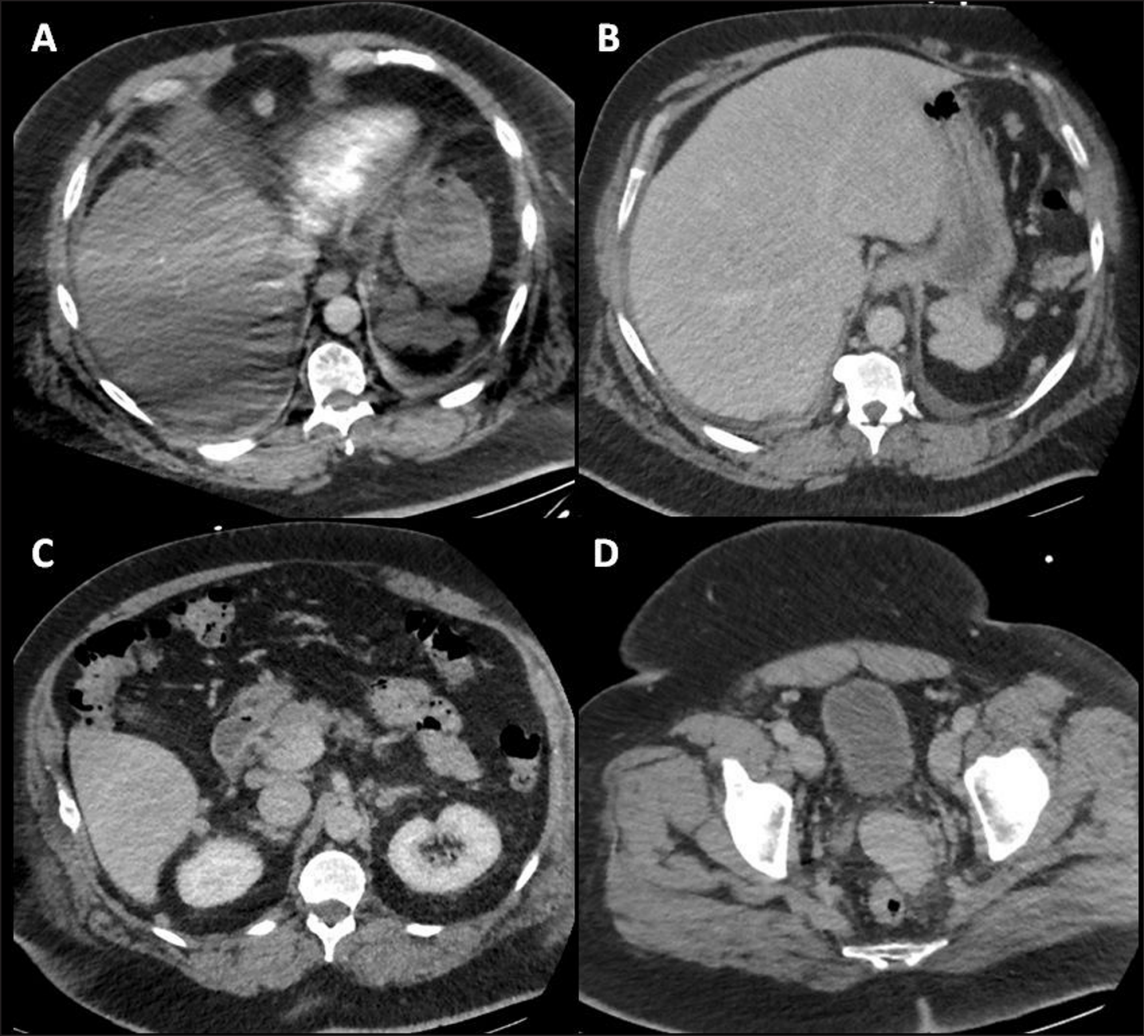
CT pulmonary angiography, arterial phase, axial slice (A) shows nodular lesions in the upper abdomen. Abdominal CT, portal venous phase, axial slice (B–D) shows multiples abdominopelvic nodular lesions.
